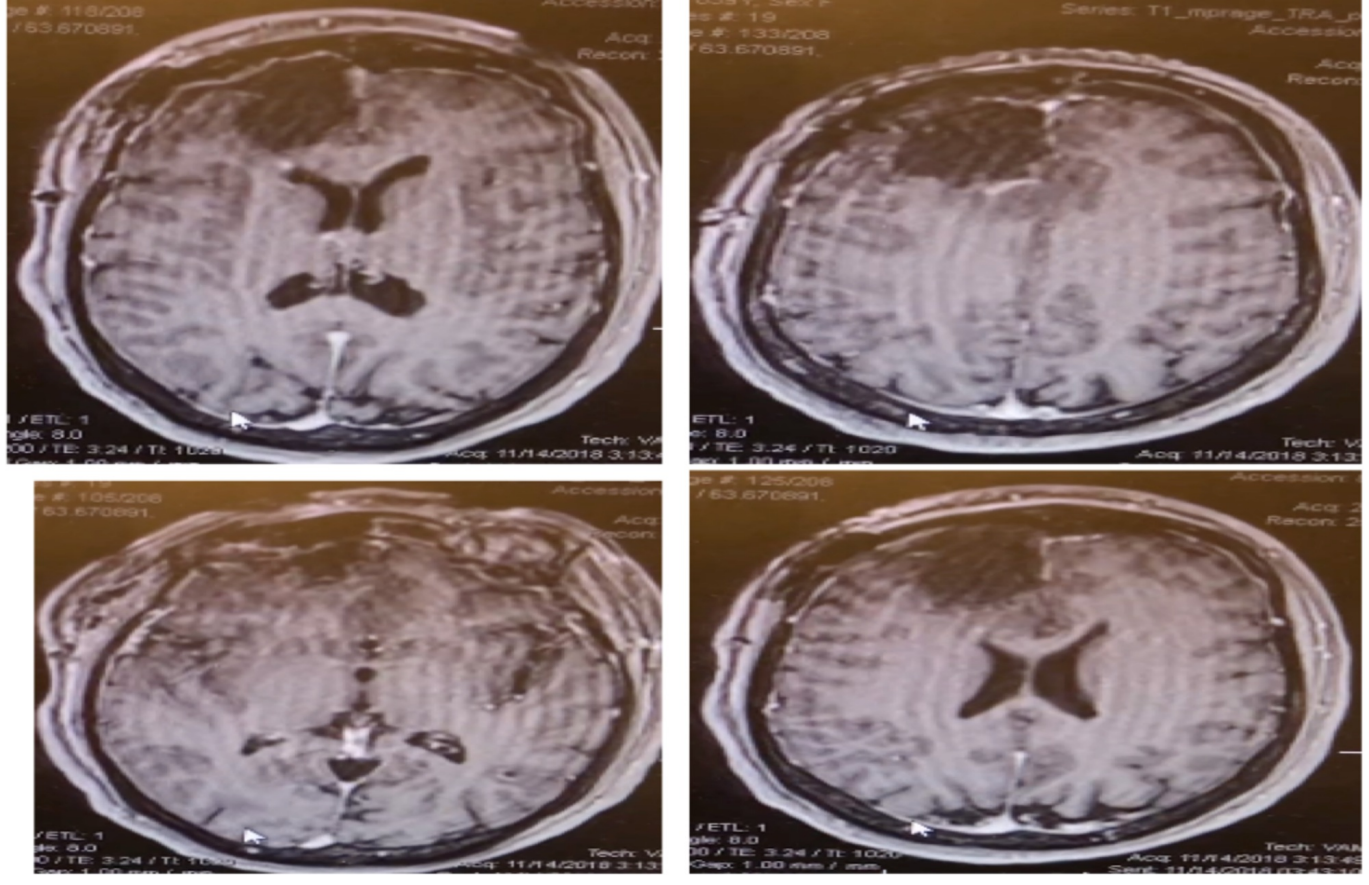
Postoperative MRI brain showing near total gross resection with tiny enhancement adjacent to right frontal horn. Images are T1 axial with contrast
